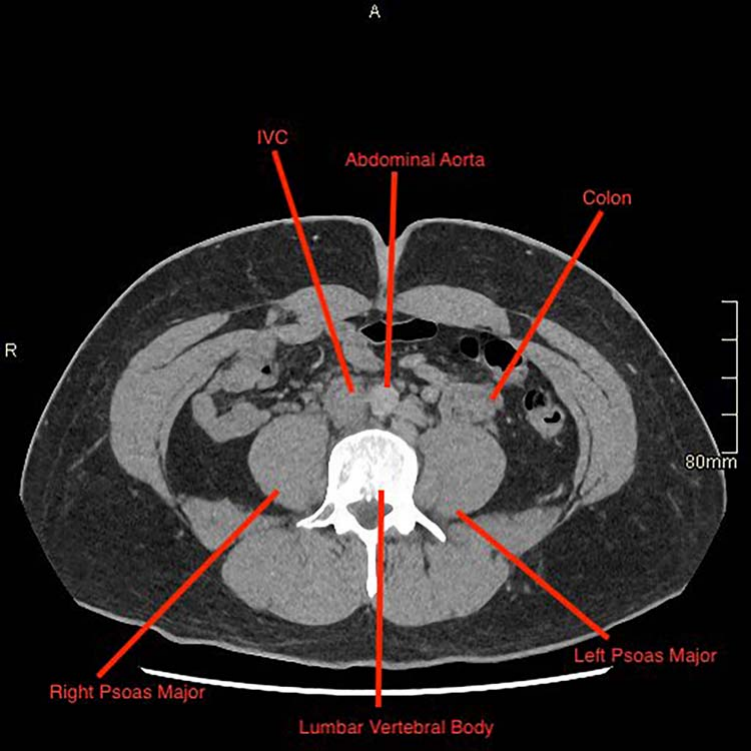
Sagittal CT image demonstrating the presence of the IVC.
